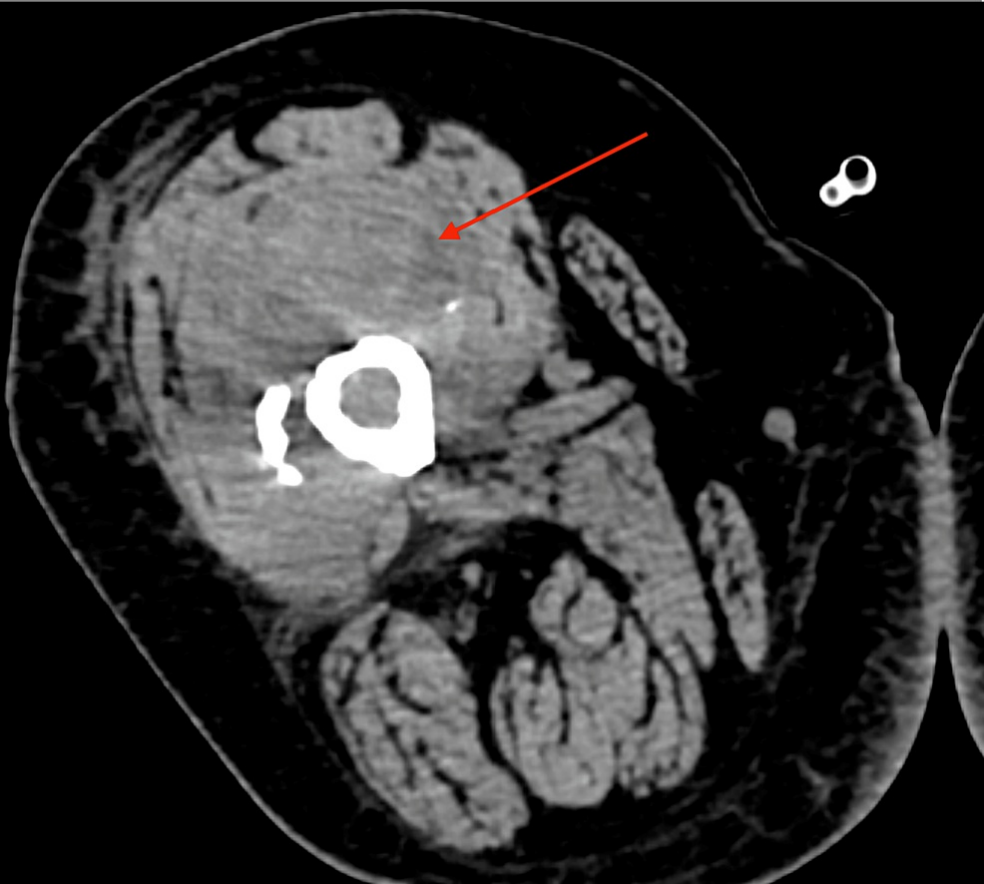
Contrast-enhanced computed tomography in axial view taken after plain film radiography demonstrated a fracture showing a mixed attenuating fluid collection anterior to the femoral fracture, suggestive of hematoma formation (red arrow).
